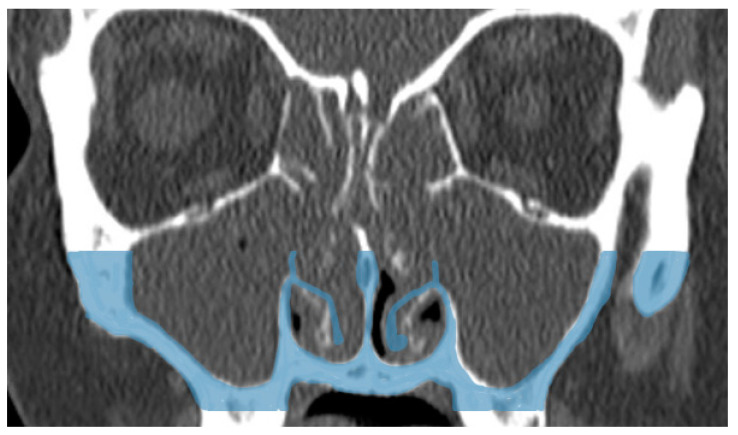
Showing merged computed tomography of pre-operative and post-operative images. Blue color shows the original position of the inferior turbinate prior to outfracturing.
